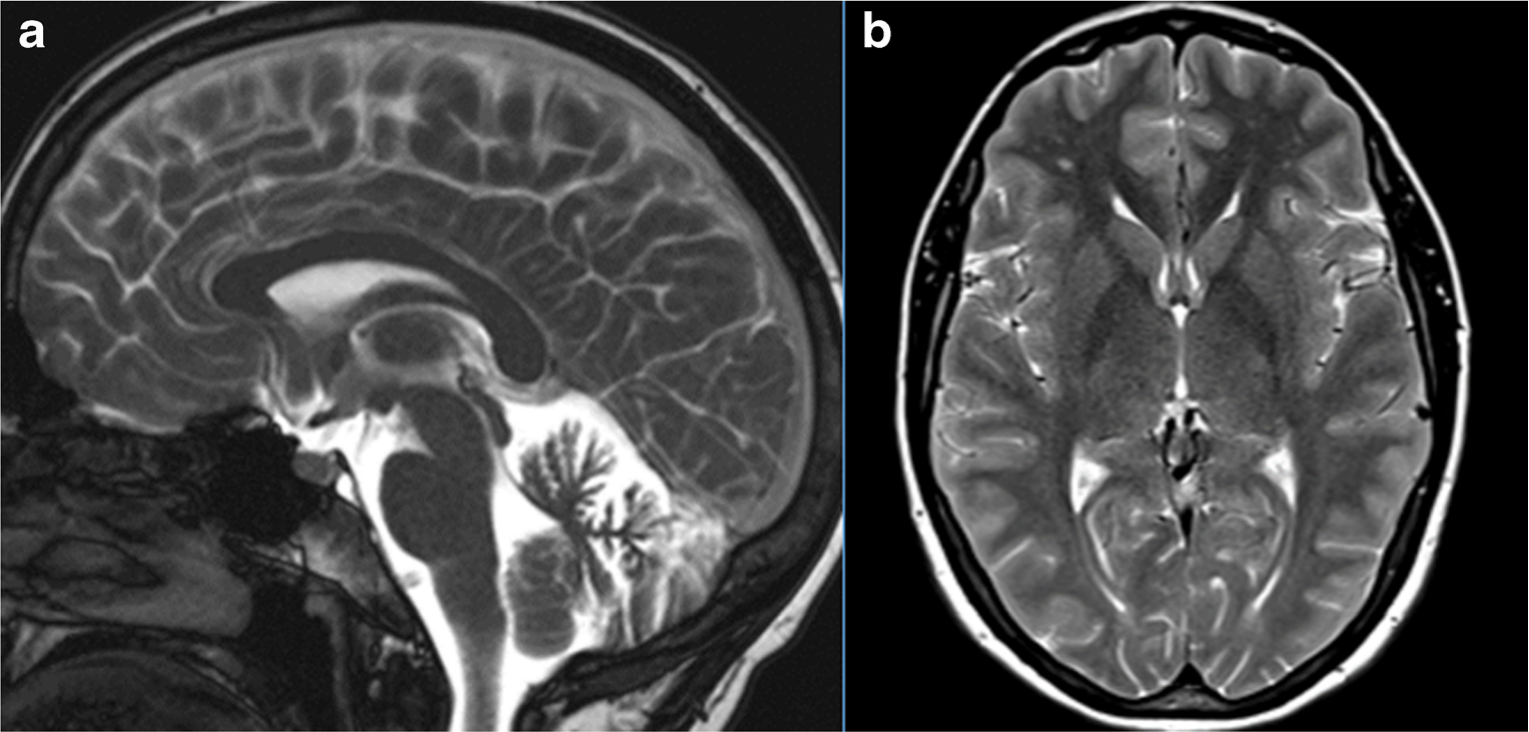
Brain MRI of patient III:2. a Midsagittal T2-weighted image shows mild vermis atrophy. b Punctate white matter hyperintensities in the frontal lobe are evident in the coronal T2-weighted image
